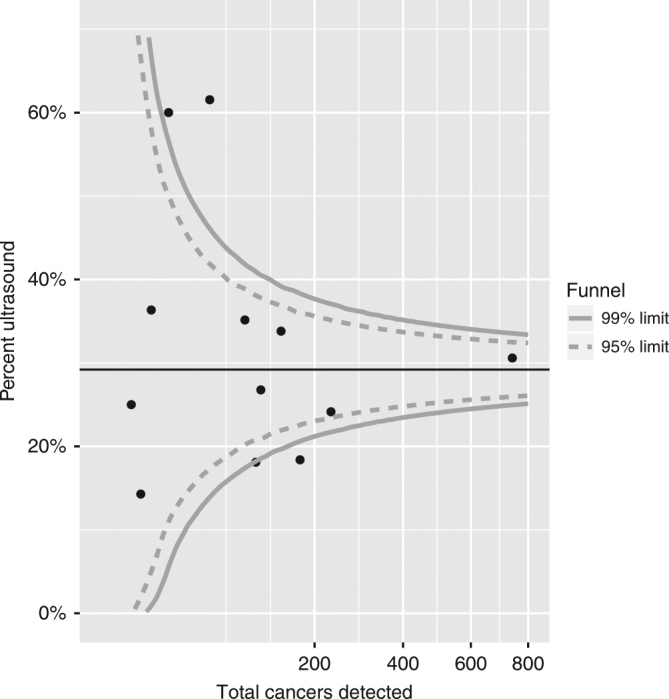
Funnel plot of the percentage of cancers detected by ultrasound against the total number of cancers detected (based on 12 studies reporting detection by both screening modalities)
